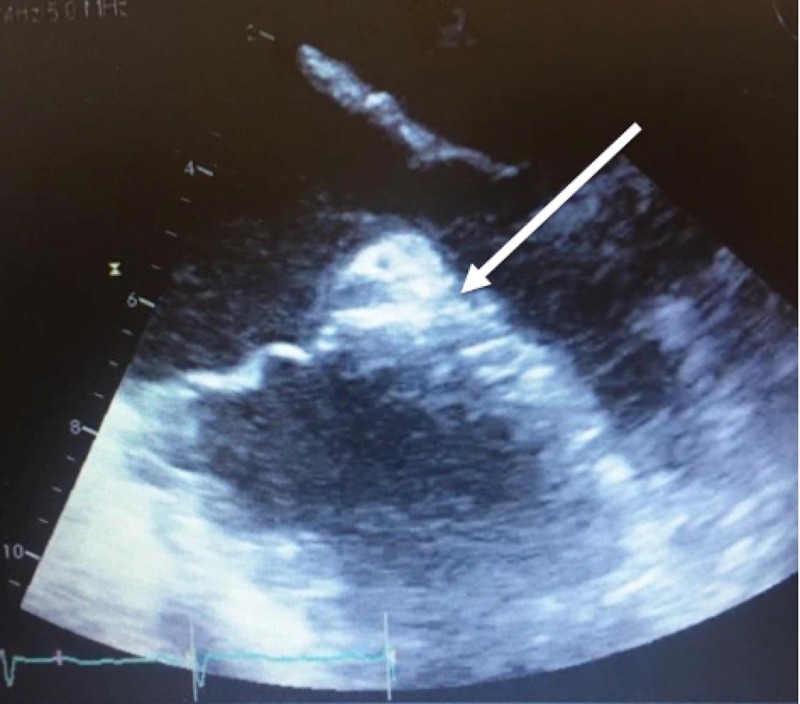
Transesophageal echocardiogram showing a large left atrial appendage (white arrow) without evidence of clot formation.
